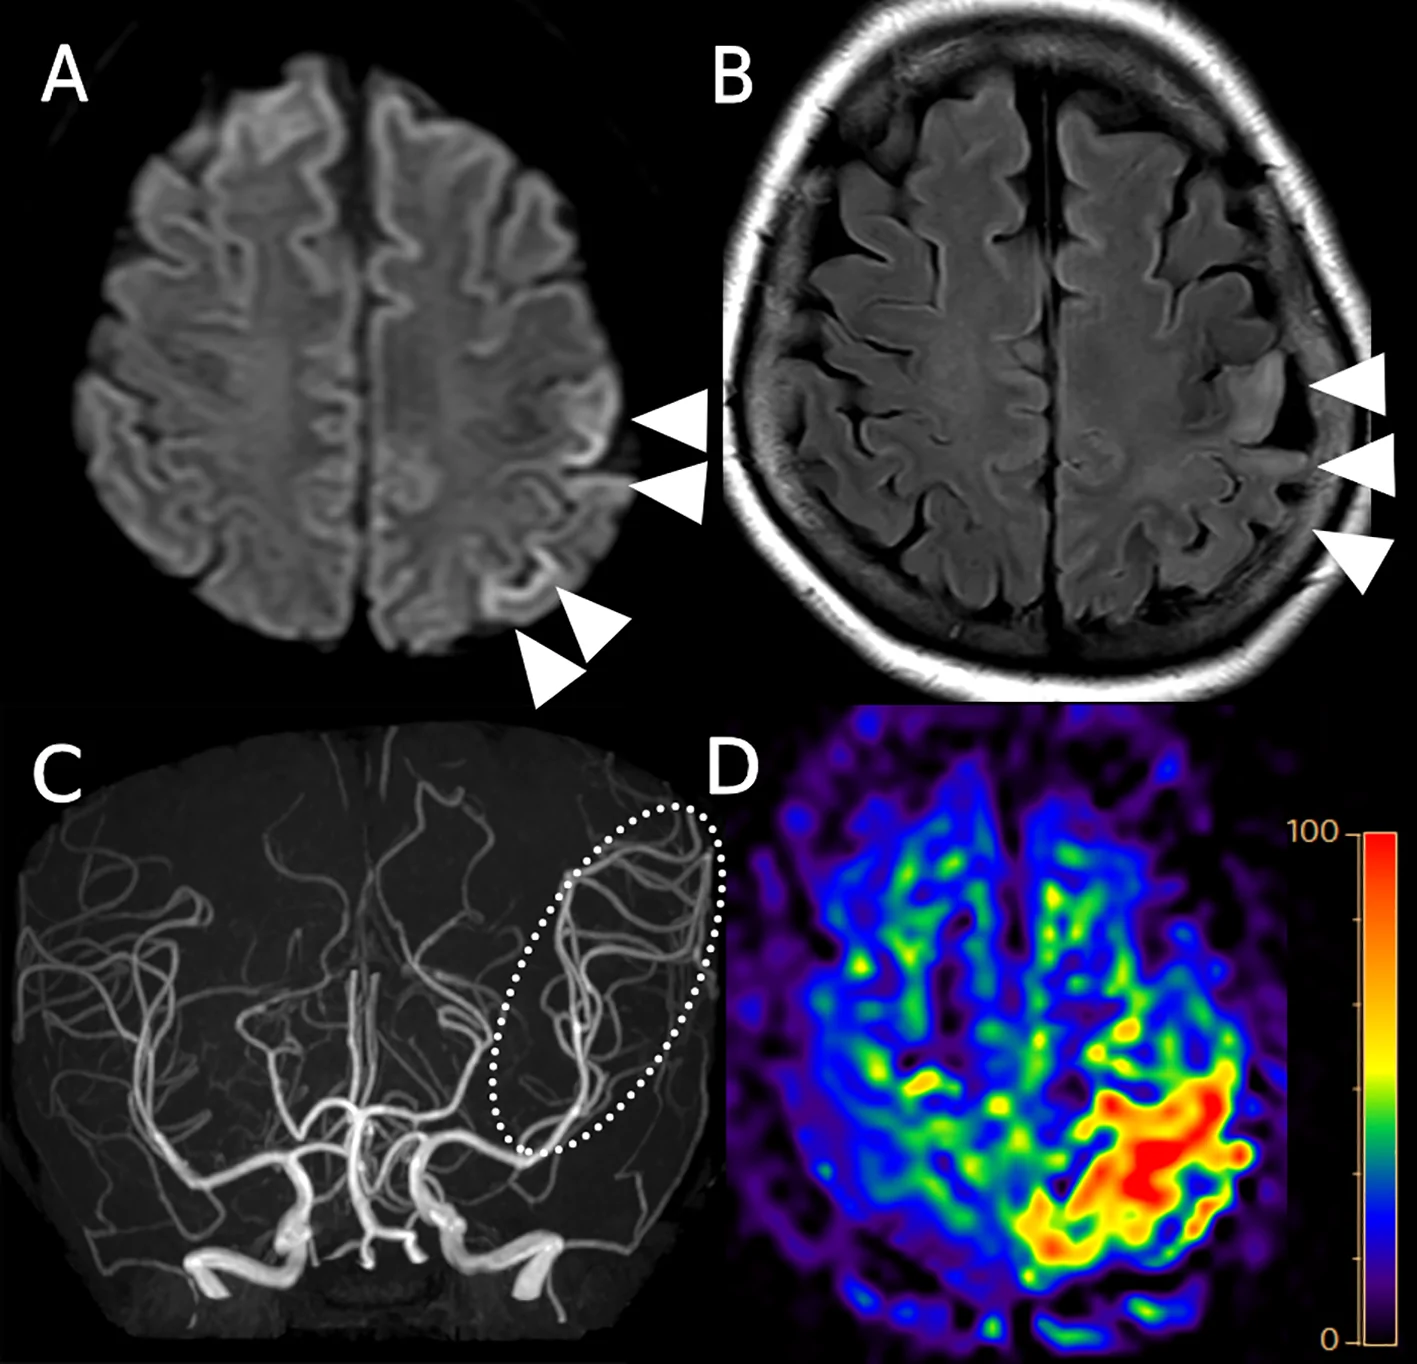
Magnetic resonance imaging findings on the second admission. (A) Diffusion-weighted imaging (DWI) shows hyperintensity along the left parietal cortex (white arrowheads). (B) Fluid-attenuated inversion recovery imaging demonstrates hyperintensity in the same region as observed on DWI (white arrowheads). (C) Magnetic resonance angiography reveals increased vascularity in the left middle cerebral artery territory (white dotted line). (D) Arterial spin labeling demonstrates hyperperfusion in the left parietal lobe.
